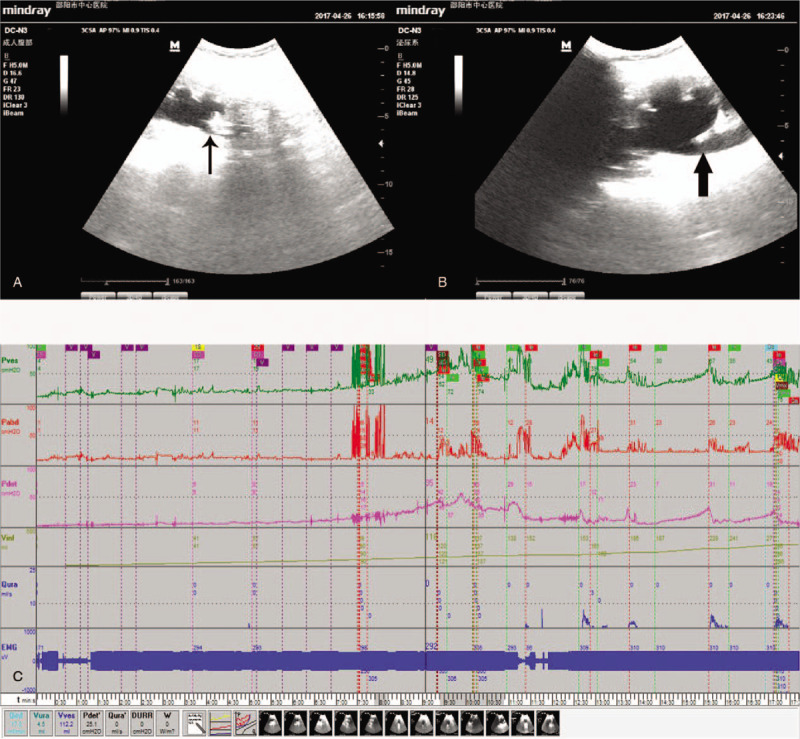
(A) Right hydroureteronephrosis (thin black arrow) was revealed at beginning of storage phase by sonography video urodynamic (VUD). (B) Aggravation of distention of right renal pelvis and ureter (thick black arrow), which indicated right vesicoureteral reflux (VUR), was detected at a low intravesical pressure (25 cmH_2_O). (C) The markedly decreased maximum bladder capacity (MBC) of about 120 mL (normal about 400 mL), at which the patient has a feeling of strong urgency desire to void, a poor bladder compliance of 9 mL/cmH_2_O (normal about 40 mL/comH_2_O), and detrusor overactivity was demonstrated by sonography VUD.
